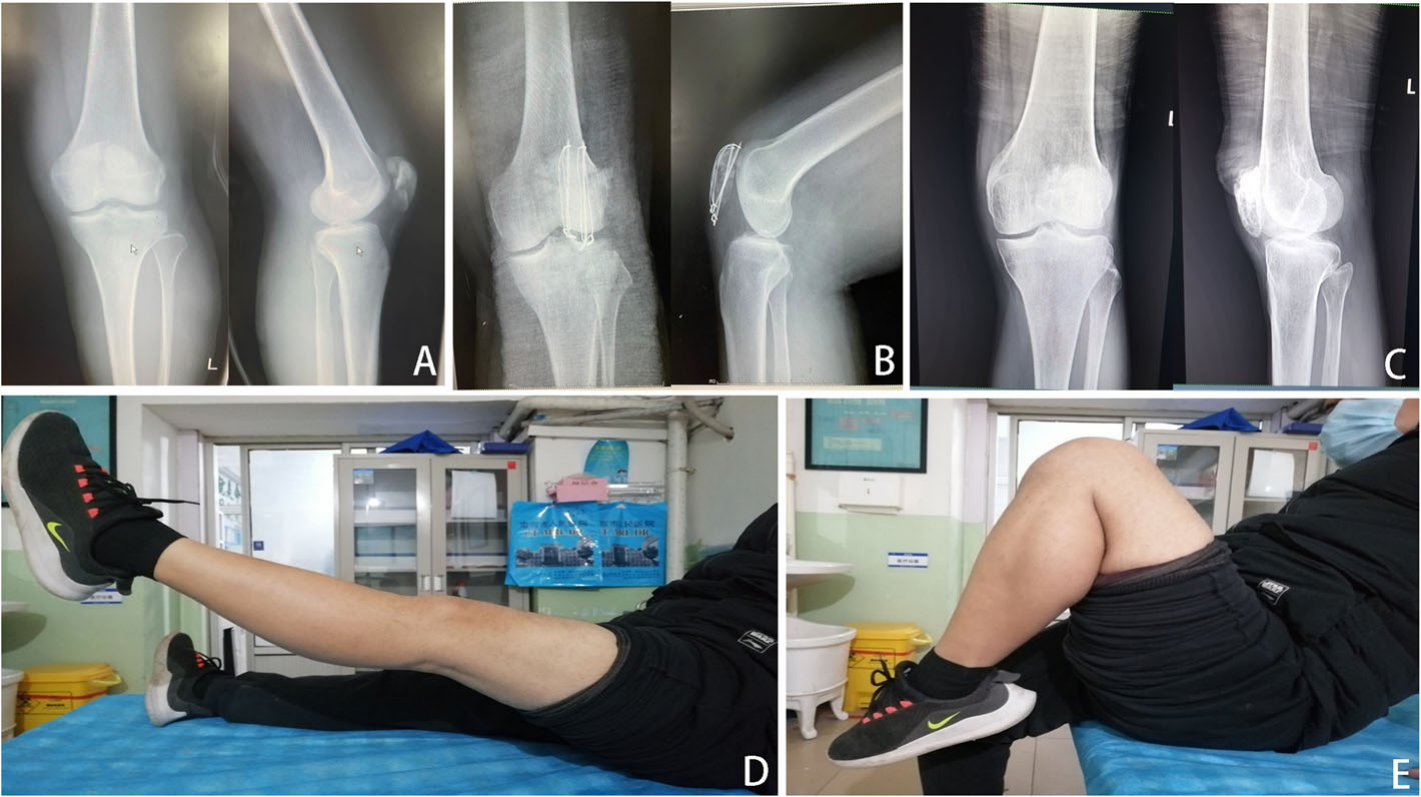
A Male, 45 years old, direct blow, anteroposterior and lateral radiographs of the left knee showed patellar comminuted fractures preoperatively and B postoperatively at 1 year, the fracture was well healed. C Anteroposterior and lateral radiographs of the left knee postoperatively at the final follow-up showed patellofemoral arthritis. D Range of motion was restored normally at the final follow-up
